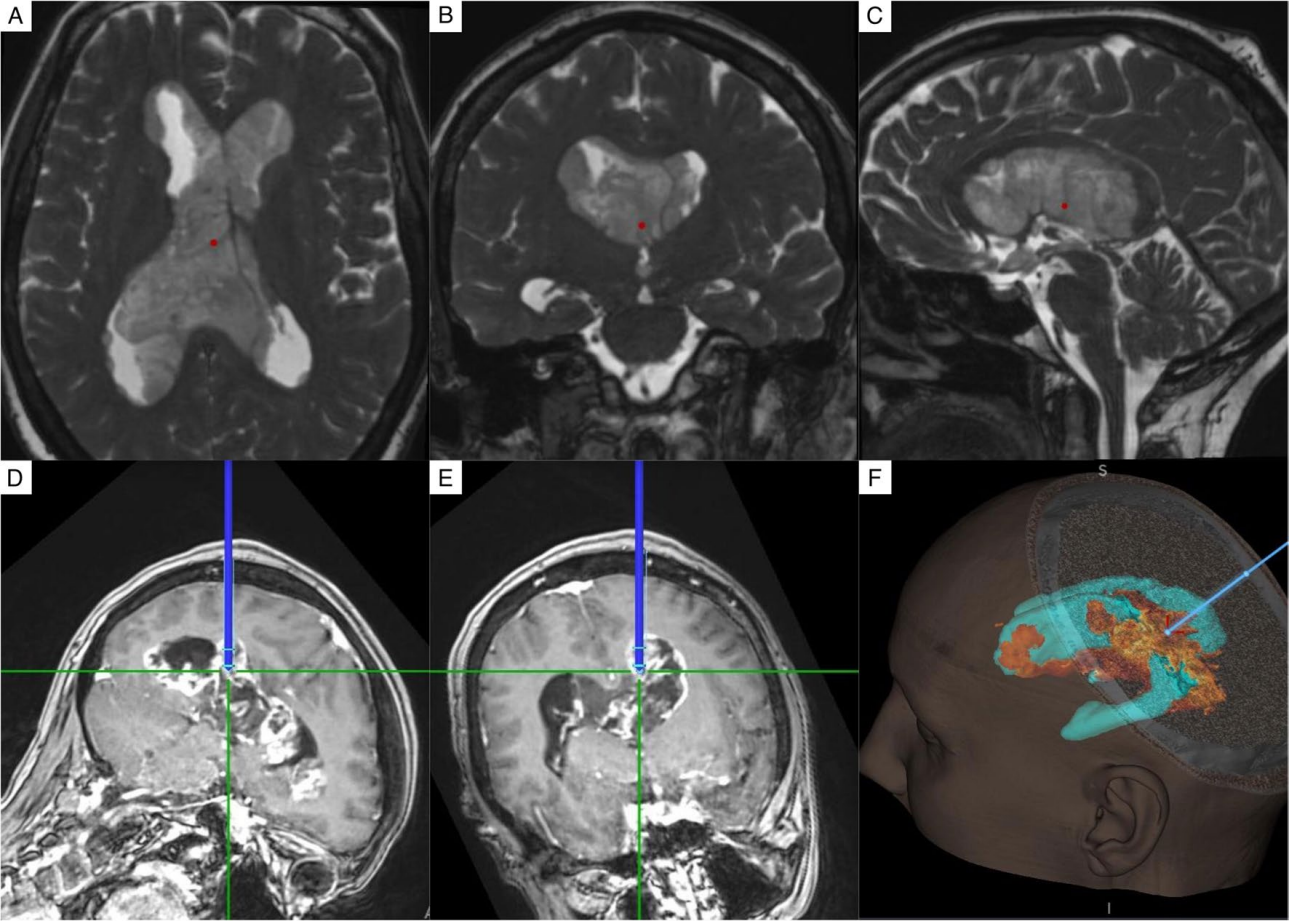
MRI appearance and stereotactic biopsy planning. A–C T2-heavy weighted high-resolution images showed a purely intraventricular hyperintense lesion compared to white matter, without brain parenchyma involvement. D, E Non-homogeneous contrast enhancement appears at T1-weighted images after gadolinium injection. F The stereotactic biopsy target was identified in the left ventricular carrefour (the lesion is depicted in orange)
